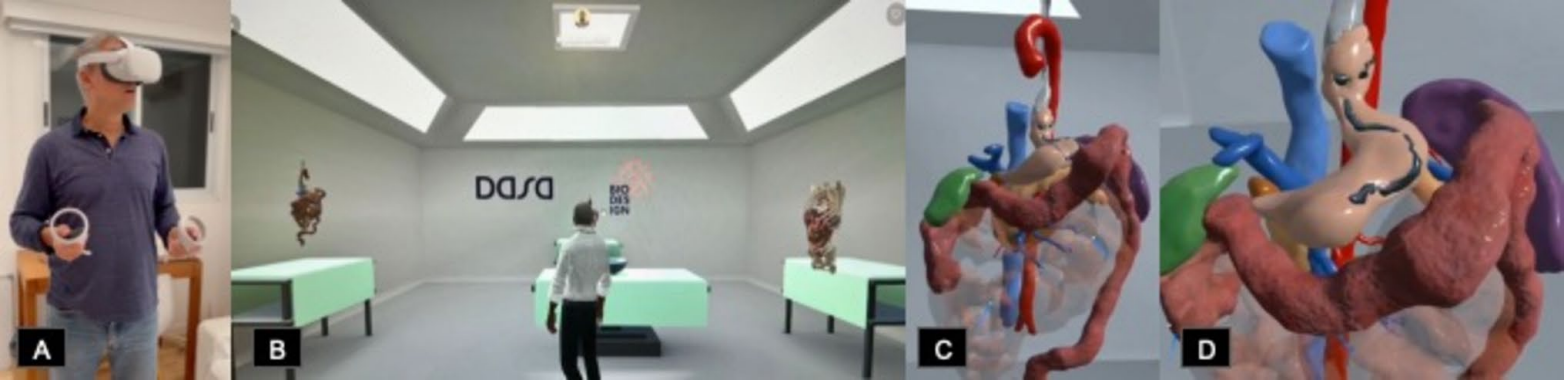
VR example. A The case discussed was a postoperative surgery for correction of a previous gastric bypass. The patient’s DICOM images from a volumetric abdominal CT were used to process the 3D visualization. A. HMD used to visualize the virtual reality. B Virtual room used to show the case for the surgeons in the metaverse. C 3D visualization of the case in a panoramic view. D Zoom in of the case during the manipulation within the metaverse, showing the area of surgical interest (previous surgical suture)
